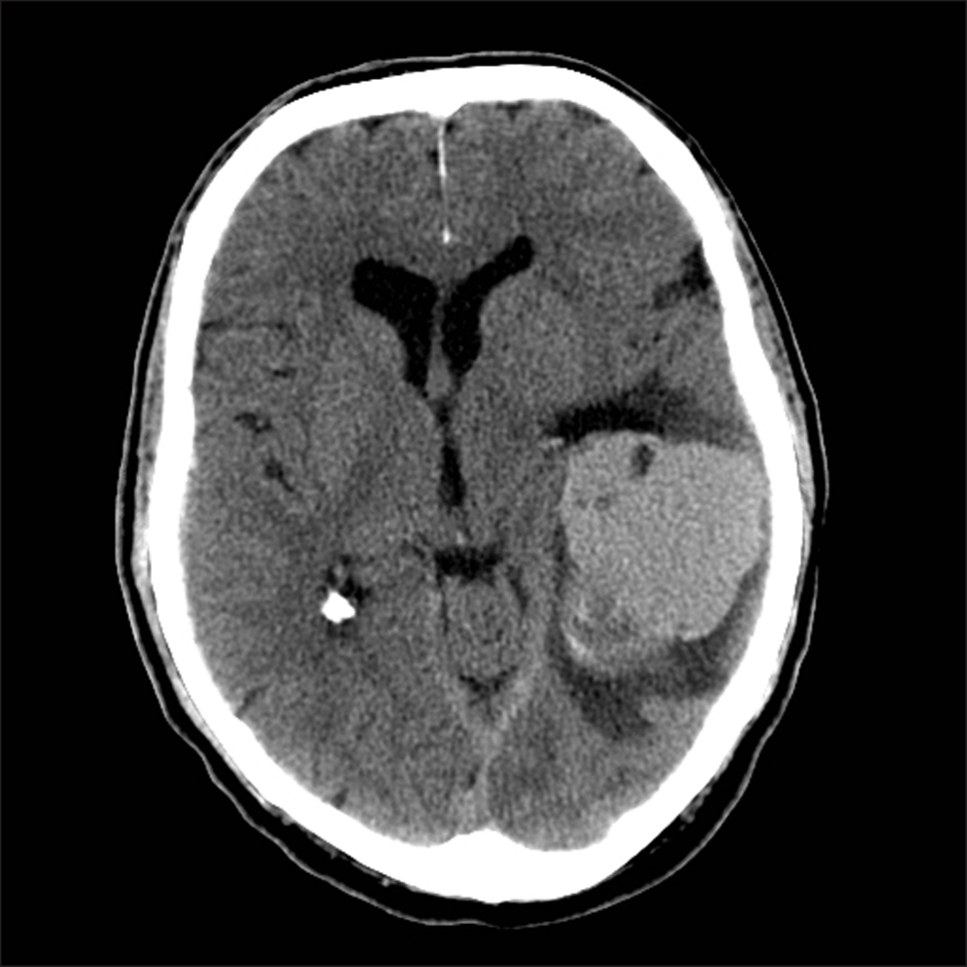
Unenhanced CT shows a well-defined, spontaneously hyperattenuating lesion in the left frontotemporal lobes. Posteriorly, a heterogeneously hyperattenuating/hypoattenuating area can be seen. Cystic/necrotic components are depicted within the lesion and vasogenic edema is surrounding the lesion.
